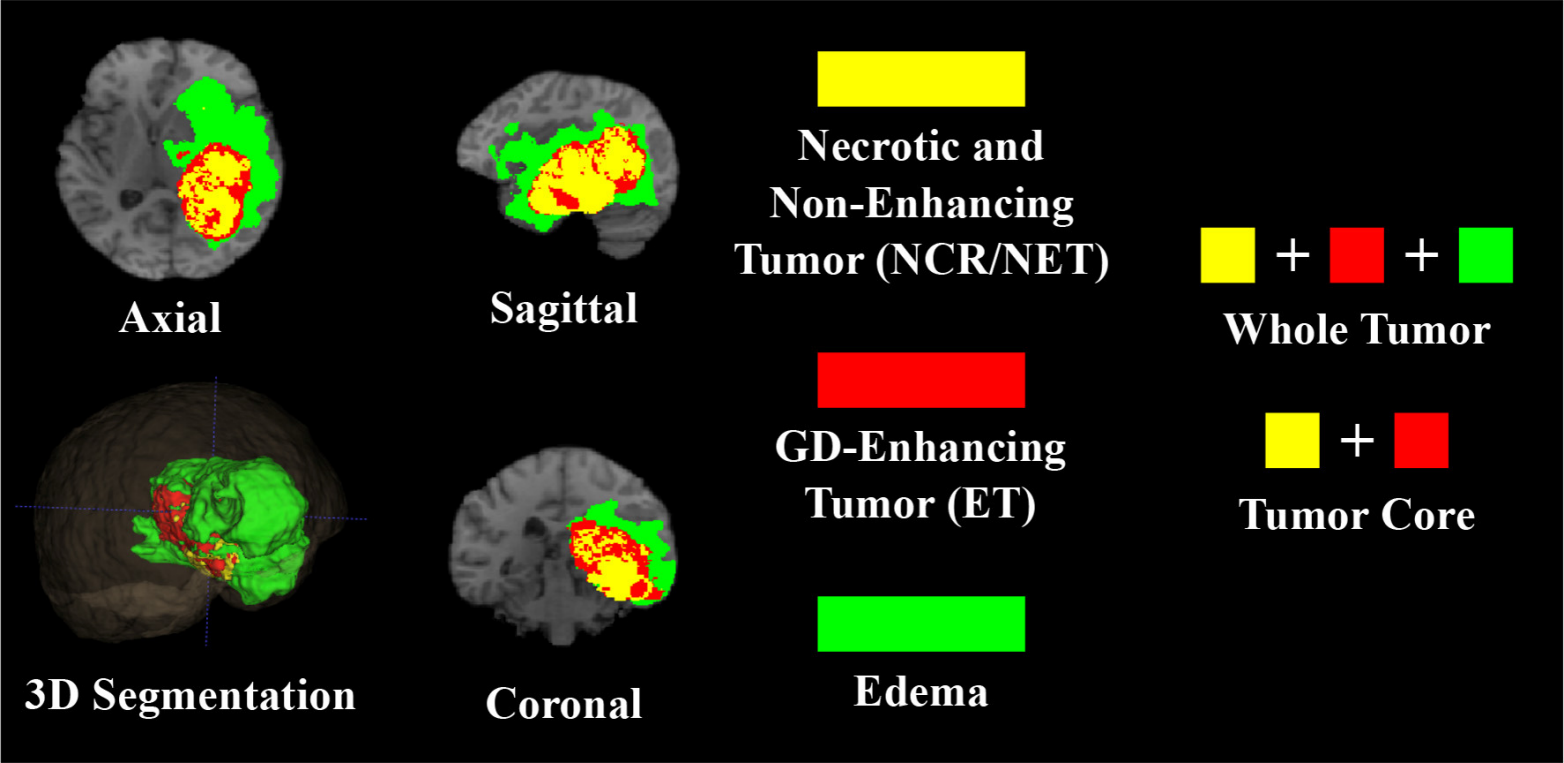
T1 MRI of a sample HGG patient with 3D segmentation of different intra-tumoral structures (ED, ET, and NCR/NET) along three principal planes (axial, sagittal, and coronal).
